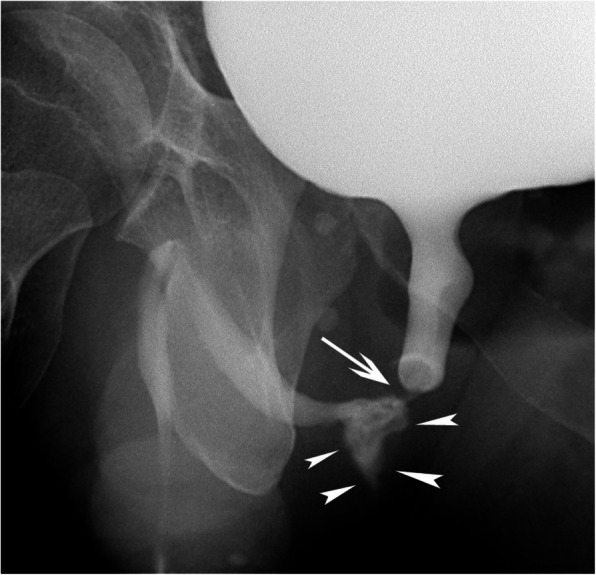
Retrograde urethrography. Oblique plain film after retrograde contrast injection clearly demonstrates the post-traumatic stricture (arrow) and the contrast extravasation (arrowheads) at the posterior part of the pars bulbosa urethrae
